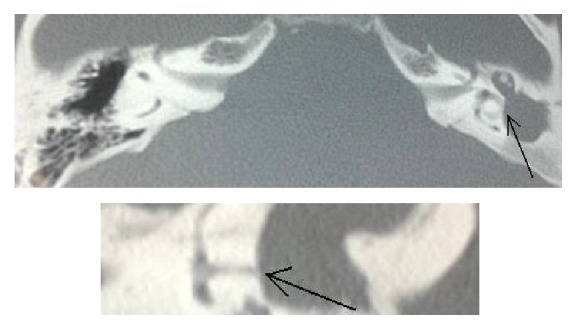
Case 1. Two different sections in the CT scan showing the fistula of the left horizontal semicircular canal and the middle ear filled with cholesteatoma and inflammatory tissue.
